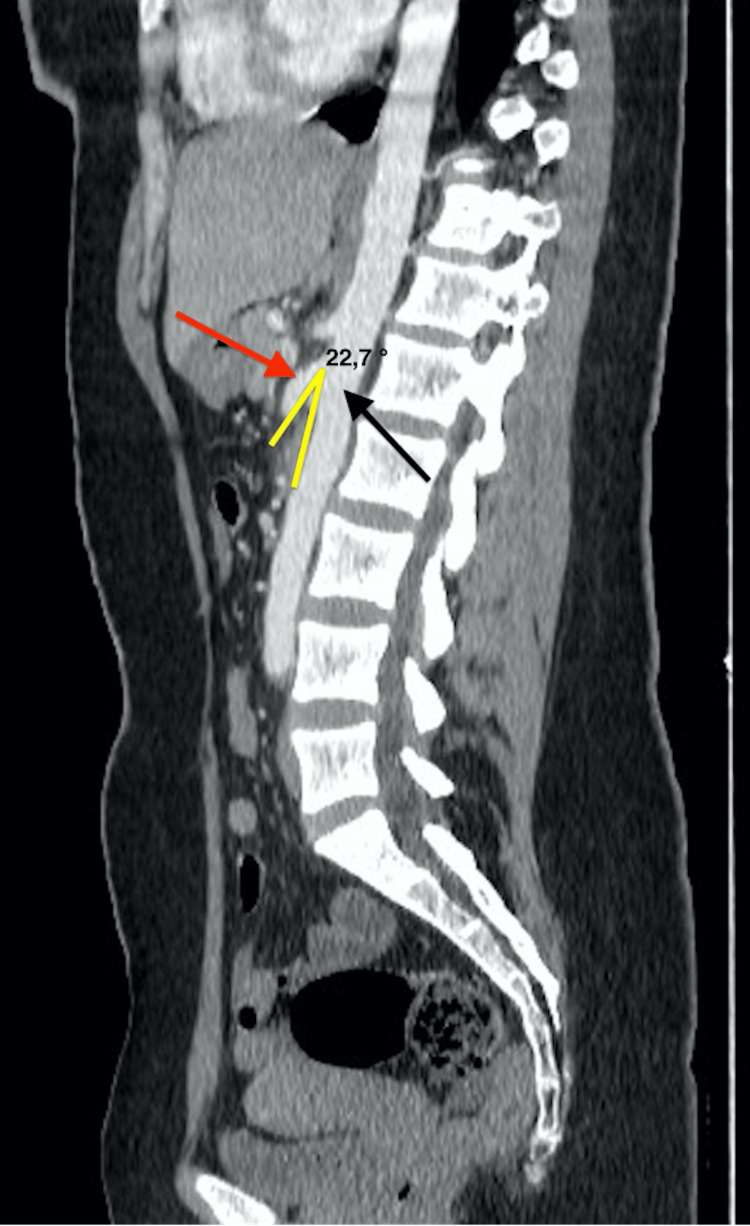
Sagittal CT angiography section showing an angulation between the abdominal aorta and the superior mesenteric artery of 22,7°. Black arrow: abdominal aorta Red arrow: superior mesenteric artery The angle between the two vessels is in yellow.
